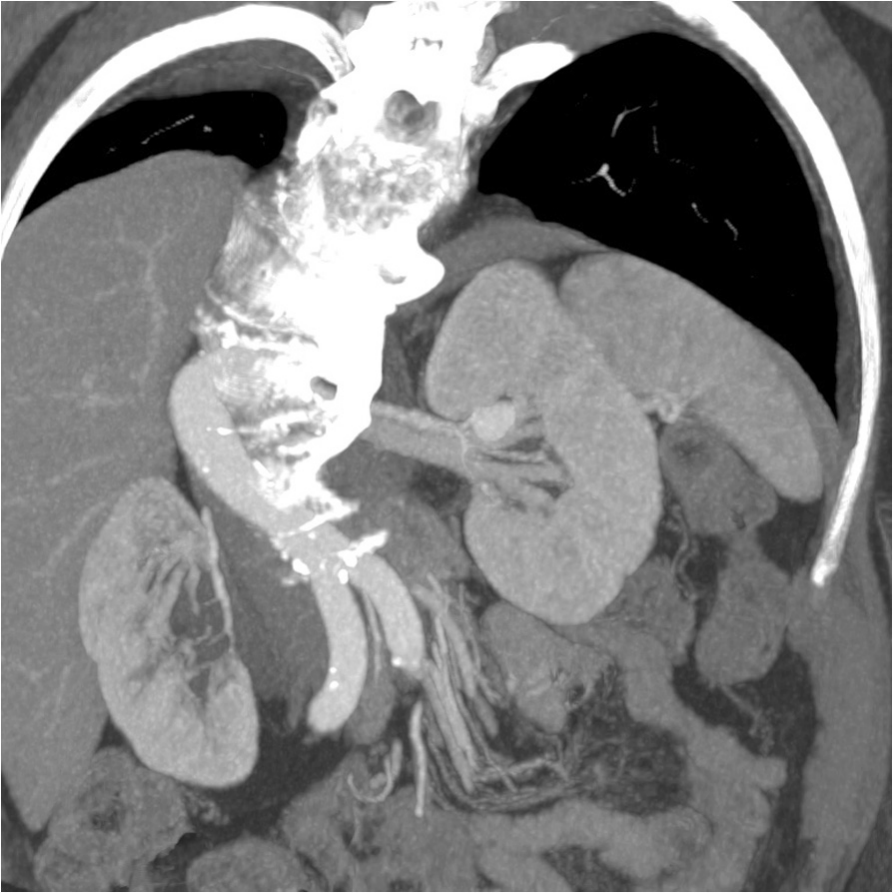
Contrast-enhanced abdominal computed tomography. Oblique coronal multiplanar reconstruction shows the aneurysm in the upper portion of the left kidney, originating from the anterior segmental artery.
